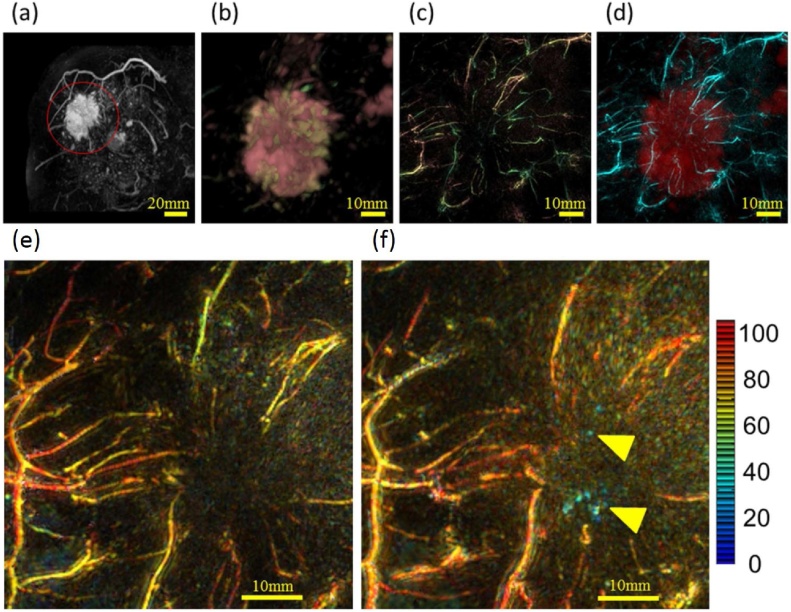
PA mammoscope images of peritumoral vasculature in IBC of a 40-year-old woman. (a) Original MR image with lesion (47 mm diameter) indicated by red circle, (b) enlarged MR image around the lesion after deforming into the shape to PA image, (c) original PA image, (d) fused PA (cyan) and MR (red) image, (e) enlarged PA image at 795 nm (signifying hemoglobin distribution) showing lesser intratumoral vessels before chemotherapy and (f) enlarged PA image at 795 nm showing increased number of finer intratumoral vessels after chemotherapy. Relative sO_2_ values indicated according to the color bar show a decrease in (f) compared to (e) indicating hypoxia (yellow arrows). Reprinted with modification from Ref. [52], image licensed under http://creativecommons.org/licenses/by/4.0/.
